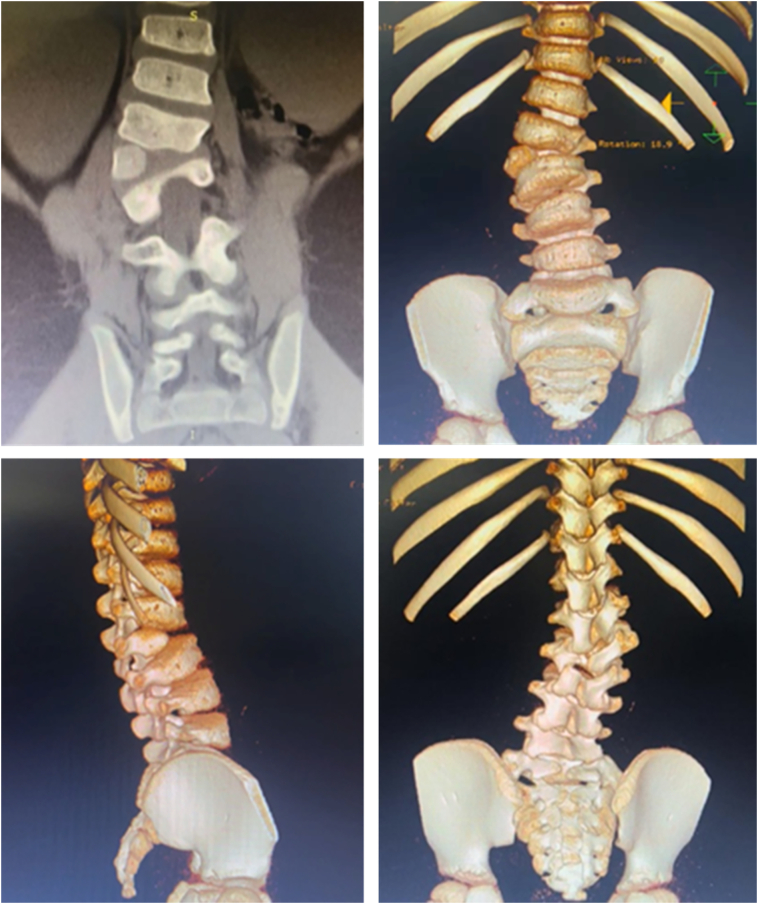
The CT-scan and 3D CT-scan 3 years post MSCs implantation. The CT showed a single fully segmented hemivertebra at lumbar level. There was no ectopic growth after implantation.
